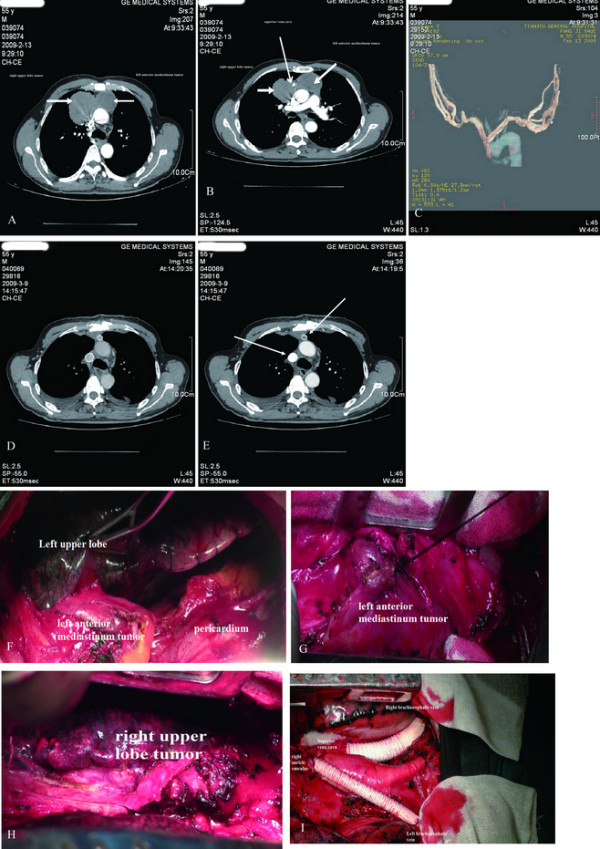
Chest computed tomographic scan. Figure 1A, 1B An enhanced Chest computed tomographic scan revealed a mass in the anterior segment of the right upper lobe with continuation to some mediastinal swelling lymph nodes. Multiple swelling lymph nodes could be found in the mediastinum. Figure C Three-D reconstruction showed the superior vena cava, whose lumen was unobstructed but deformated under the compression of the mass. Figure 1D, 1E Postoperative enhanced Chest computed tomographic scan images. Figure 1F, 1G Surgical findings of the mediastinal mass. Figure 1H Surgical findings of the intrapulmonary mass. Figure 1I Reconstruction of left brachiocephalic vein to right auricle and reconstruction of right brachiocephalic vein to superior vena cava.
